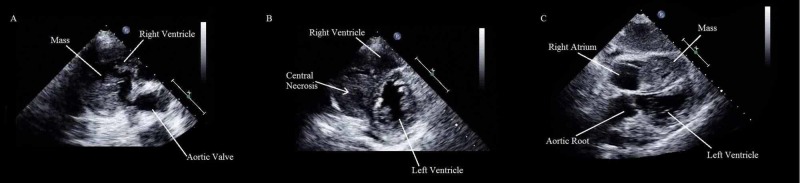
Transthoracic echocardiography A. Short-axis, great vessels off-axis view, showing a lobulated, cerebriform mass in the right ventricle near to the outflow tract. B. Short-axis, middle ventricle view, showing a mass occupying the right ventricle, adherent to the interventricular septum, with an anechoic central core. C. Subcostal view, showing a mass, with multiple anechoic foci, occupying a large amount of the right ventricle.
